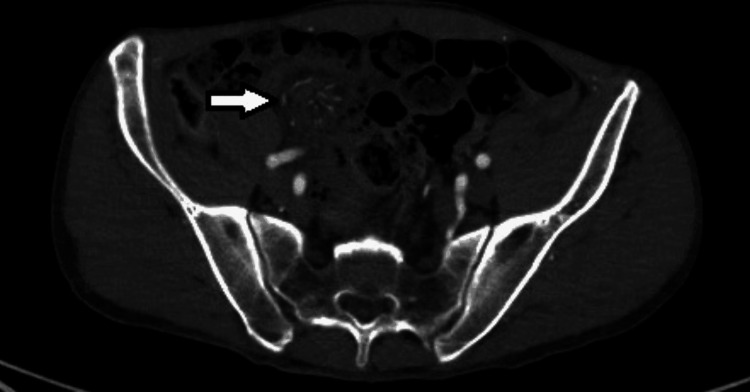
CTA abdomen pelvis demonstrating swirling of the mesentery (white arrow) CTA, CT angiography
